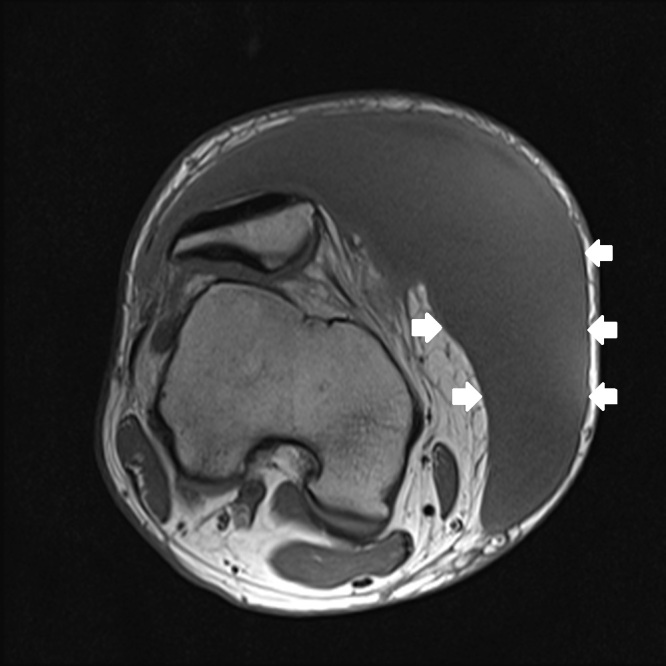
Axial T1-weighted magnetic resonance imaging shows enhancement of the capsule.
